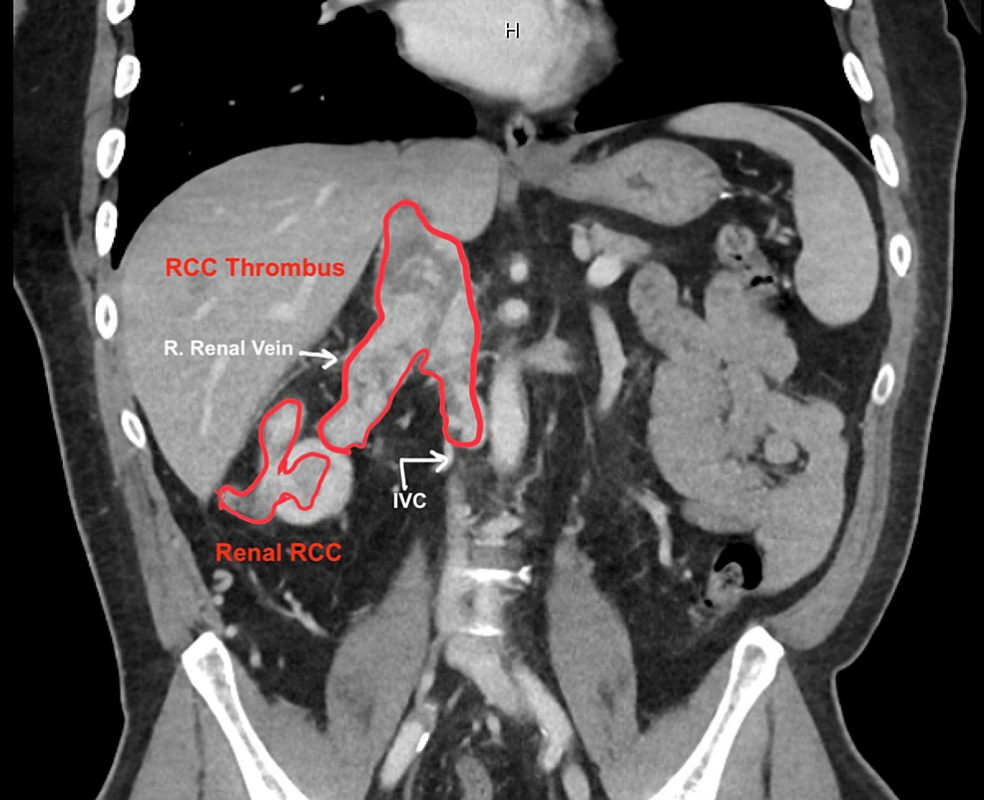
CT abdomen (coronal view) demonstrating right kidney renal cell carcinoma with tumor thrombus in the right renal vein extending to the inferior vena cava. RCC: Renal cell carcinoma; IVC: Inferior vena cava
